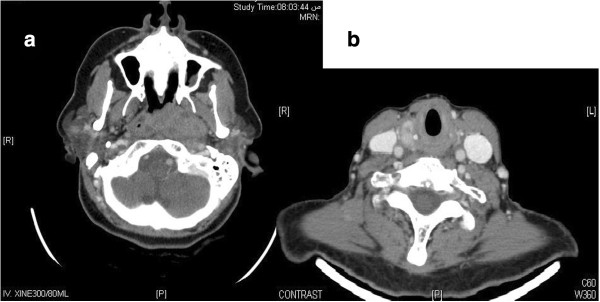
CT showing (a) Left sided nasopharyngeal tumor infiltrating into the left parapharyngeal space and abutting the left internal carotid artery and (b) Thyroid nodule right lobe.
